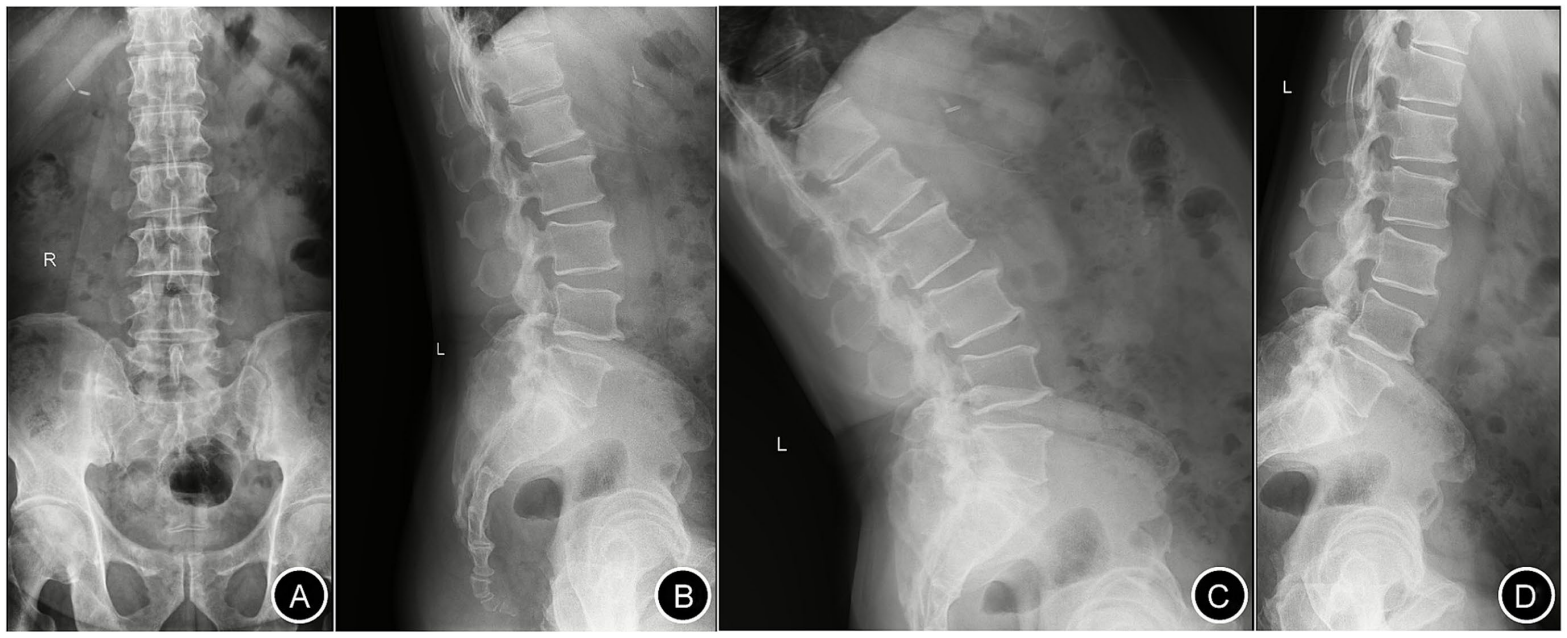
Lumbar spine X-ray. Panels (A–D) represent the anteroposterior, lateral, hyperextension, and hyperflexion views, respectively.
